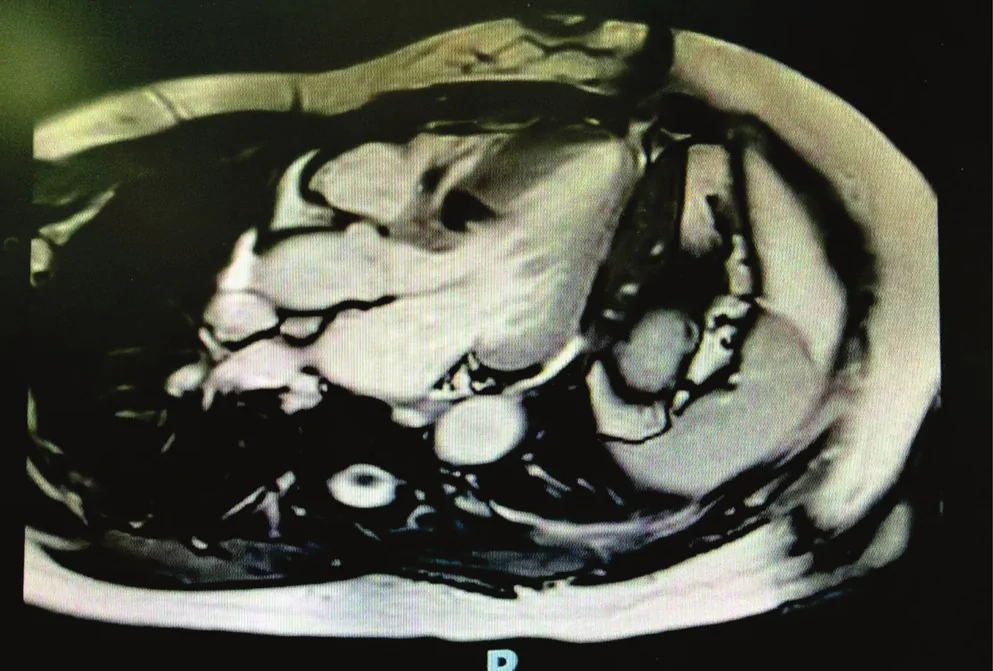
Cardiac MRI (gadolinium‐enhanced) showed focal late gadolinium enhancement (high signal intensity) in the interventricular septum 1 week post‐ablation, consistent with post‐ischemic myocardial scarring (arrows). This imaging finding correlates with ethanol‐induced necrosis of the septal perforator arteries supplying the conduction system.
